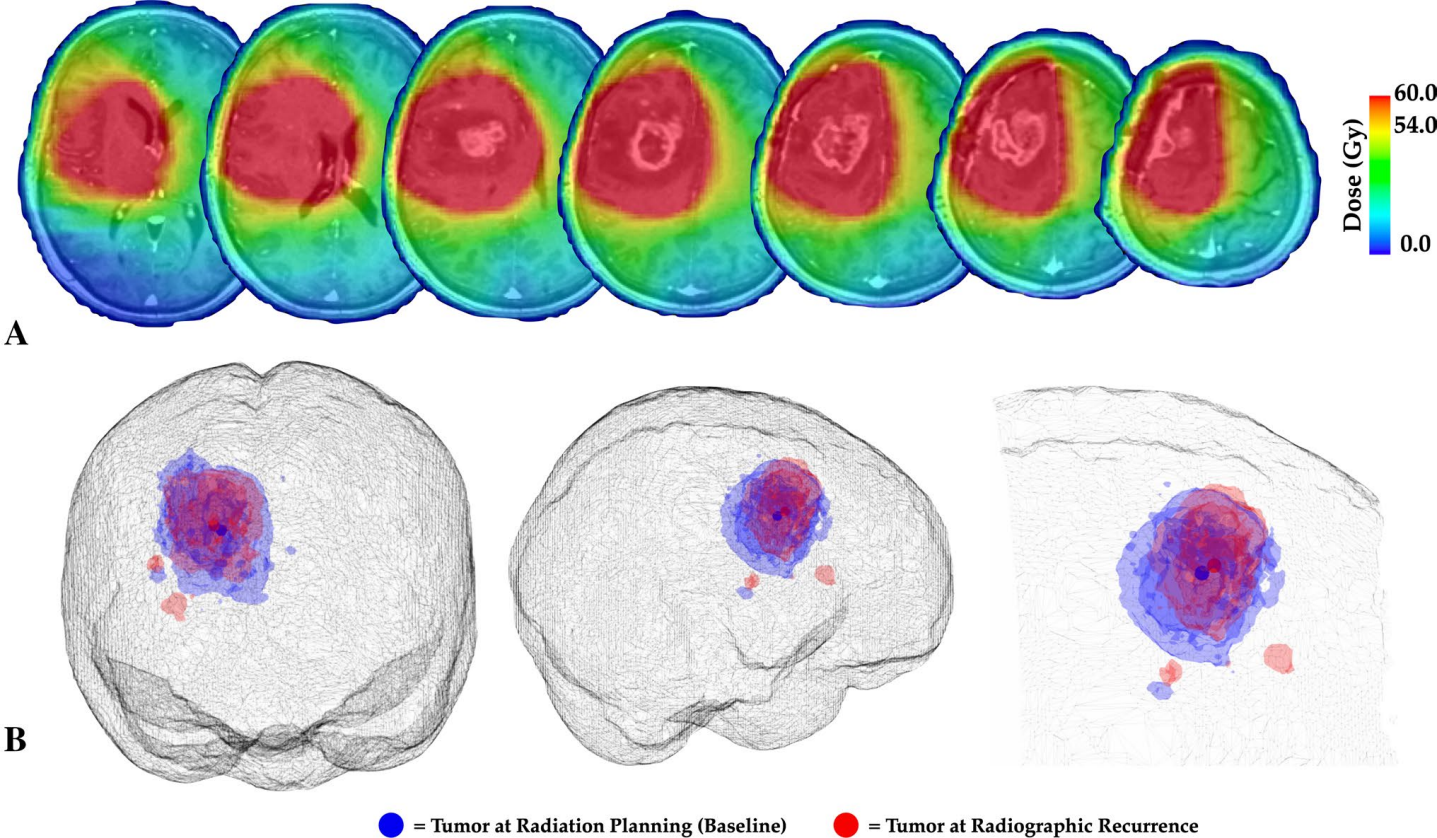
A Fusion of radiation treatment dose planning with pre-radiation MRI scans. B Segmented enhancing tumor at baseline (blue) and at the time of radiographic recurrence (red) as well as the centroid location for both (solid spheres in center of enhancing regions of interest)
